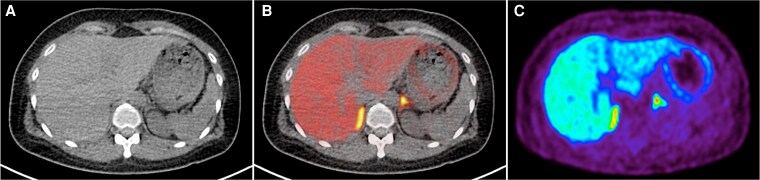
^11^C-MTO PET-CT for case 4—no adrenal lesion seen on CT imaging (A). AVS showed clear left lateralization, but MTO detected a left adrenal nodule with only SUVmax 45.2, compared to SUVmax of 39.5 over the right adrenal body on the fused PET-CT imaging (B) and PET imaging (C). The SUVmax ratio of left adrenal nodule-to-contralateral gland was 1.14 (less than cutoff of 1.25 to define lateralization). Abbreviations: ^11^C-MTO PET-CT, ^11^C-metomidate positron emission tomography-computed tomography; AVS, adrenal vein sampling; SUVmax, maximum standardized uptake value.
